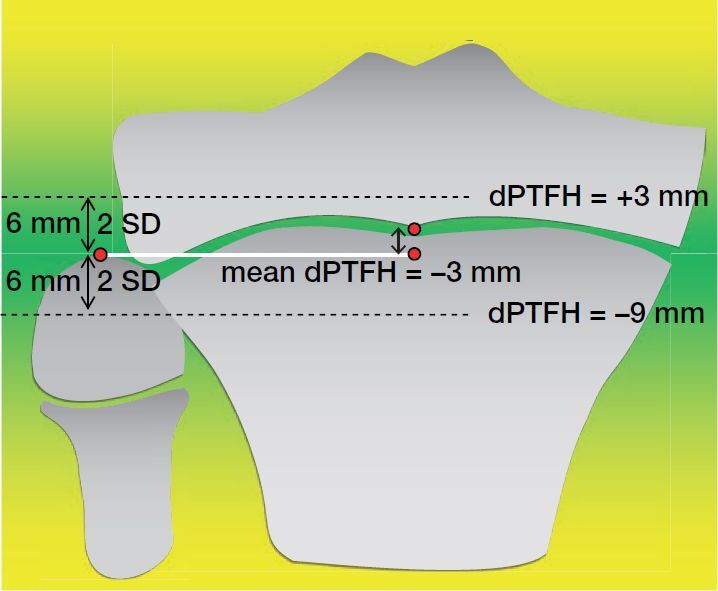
This study provides dPTFH as a new standard radiographic reference defining the anatomical localization of the fibular head in relation to the center of the proximal tibial growth plate. The mean dPTFH in children and adolescents (8–16 years) is –3 mm with an SD of 3 mm. We propose to consider deviations of dPTFH greater than 2 SD as fibular overlength (dPTFH > +3 mm) or shortening (dPTFH < –9 mm) respectively.
